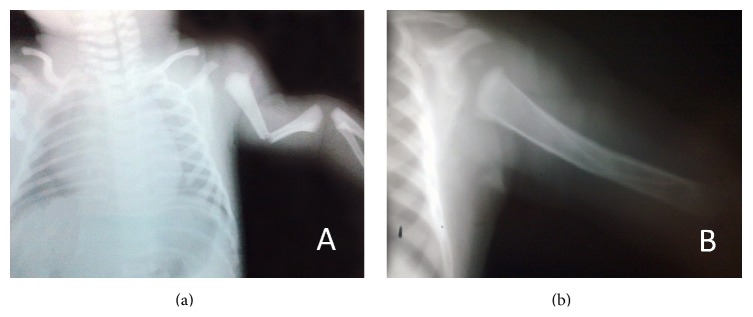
(a) Radiograph of fractured humerus prior to reduction and immobilization. (b) After 2 months.
